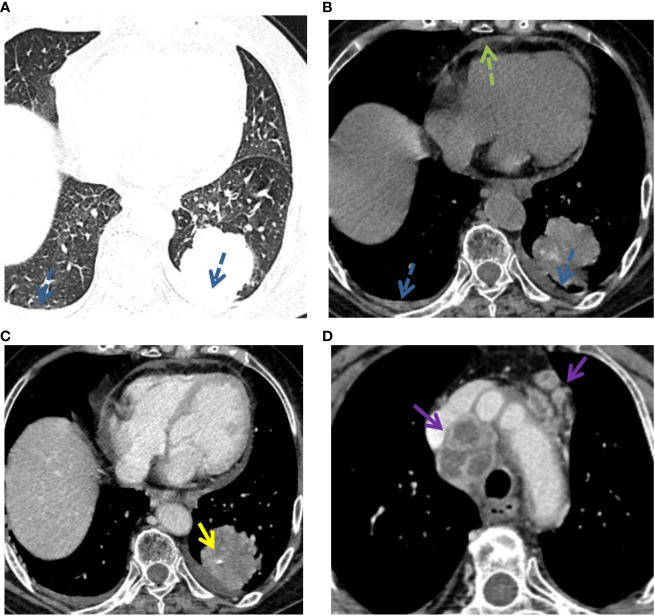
P-LADC in a 77-year-old man with cough and hemoptysis for 12 days. (A) Axial lung-window HRCT showed a localized consolidation in the left lower lobe and bilateral pleural effusion (blue dashed arrow). (B) Mediastinum window showed bilateral pleural effusion (blue dashed arrow) and pericardial effusion (green dashed arrow). Arterial phase indicated (C) an angiogram sign (yellow arrow) within the lesion and (D) multiple enlarged lymph nodes with necrosis in the mediastinum (purple arrow). HRCT, high-resolution computed tomography; P-LADC, pneumonic-type lung adenocarcinoma.
